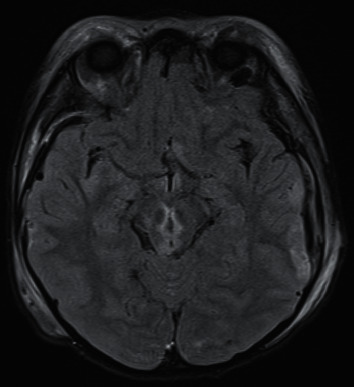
Axial FLAIR MRI showing multiple injuries in the tegmentum, tectum, and periaqueductal parenchyma of the midbrain, with involvement of the right cerebral peduncle, the right side of the pons, and the right middle cerebellar peduncle.
